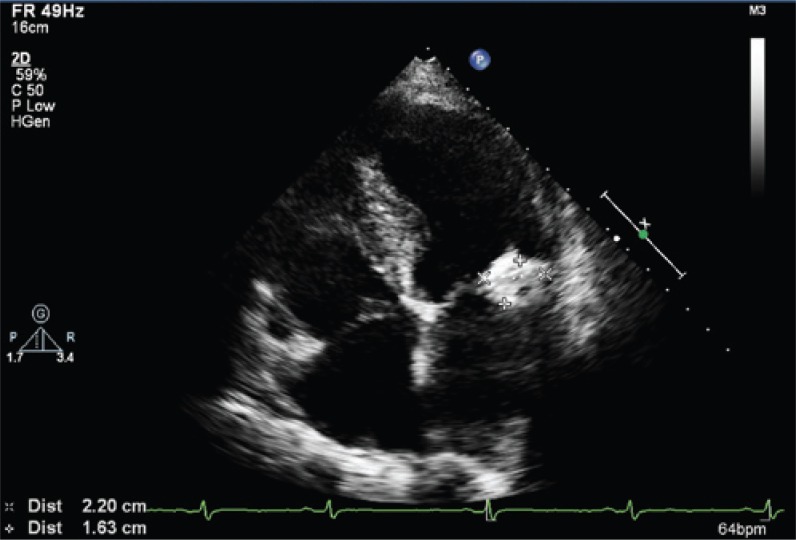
Apical 4-chambers view showing the mass located in posterior mitral valve annulus
